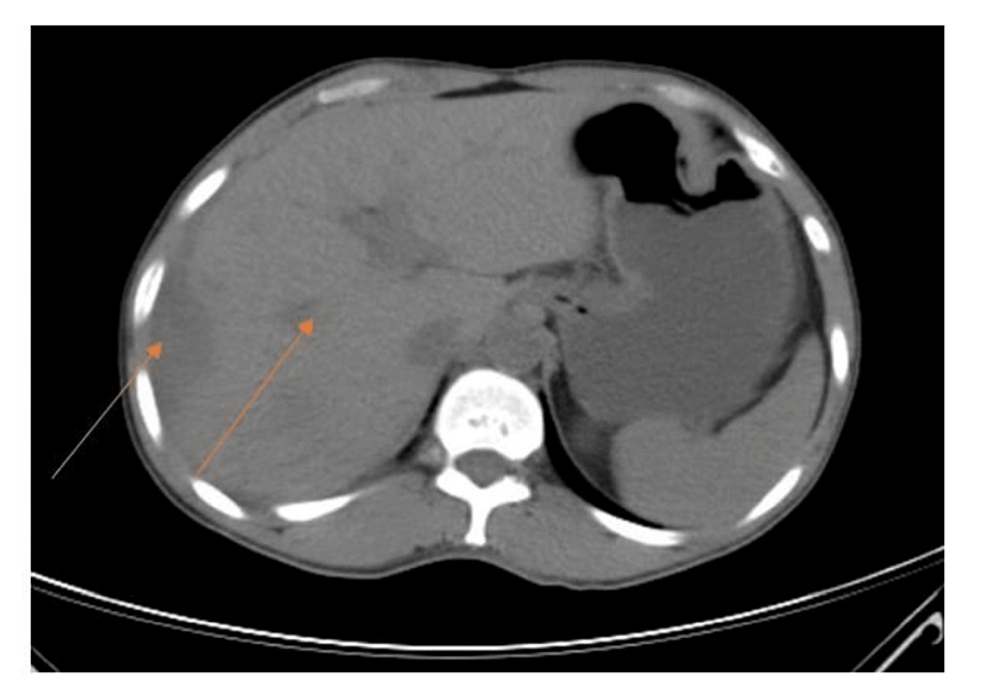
CECT abdomen and pelvis The image shows small organized abscesses in segment VI of the liver with adjacent mild fibrosis and scarring. No acute collections amenable to aspiration are seen in the present scan (shown by the arrows) CECT: contrast-enhanced computed tomography
